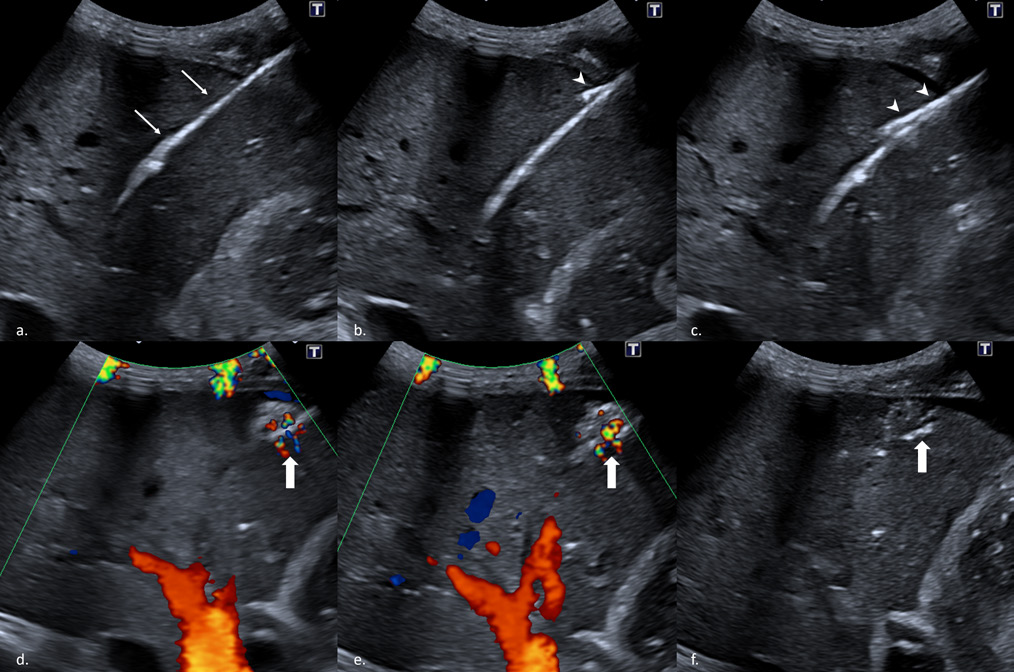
Puncture tract embolization technique. a.Ultrasound-guided identification of the puncture tract. The sheath is clearly visible (thin arrow). b and c. The microwave probe (arrowhead) is inserted along the puncture tract under ultrasound guidance and advanced 3 cm into the liver parenchyma. d and e. Ablation is performed under ultrasound guidance with 65W for 2 min, standard ablation parameters, to avoid ablation of soft tissue (thick arrow). f. Ultrasound is performed after removal of the material showing a triangular subcapsular ablation zone (thick arrow).
